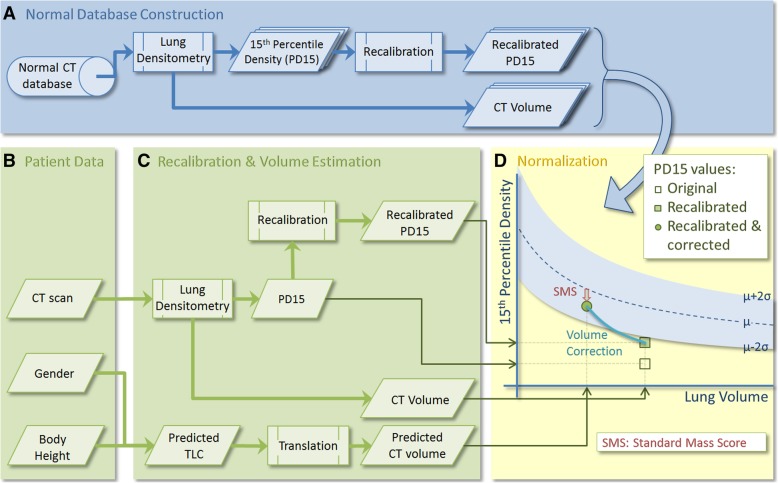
Overview of the proposed integrated method. a Construction of the normal database, producing lung volumes and recalibrated density values from a normal population; b Input data from an emphysema patient to be evaluated; c Calculation of recalibrated density data and predicted CT lung volume of a patient; d Normalization and volume correction of the density values, resulting in a Z-score for lung tissue mass: Standard Mass Score (SMS). In this particular example, a seemingly abnormally low lung density (PD15) can actually fall within the normal range for tissue mass, after accounting for different CT scanners (i.e. recalibration of lung density values) and lung volumes (volume correction)
